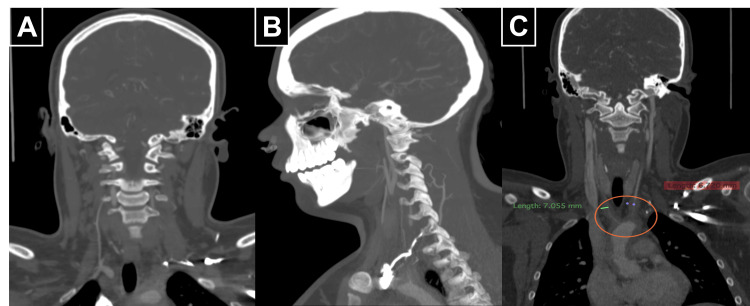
Contrast-enhanced computed tomography showing wall thickening and the truncation of supra-aortic vessels (A) Coronal view showing the abrupt truncation of the great vessels with loss of opacification beyond the aortic arch. (B) Sagittal reconstruction revealing the concentric inflammatory wall thickening of the ascending aorta and cervical arteries. (C) Coronal view with multiplanar measurements confirming the mural thickening and near-complete occlusion of the brachiocephalic trunk and left common carotid artery (orange circle).
